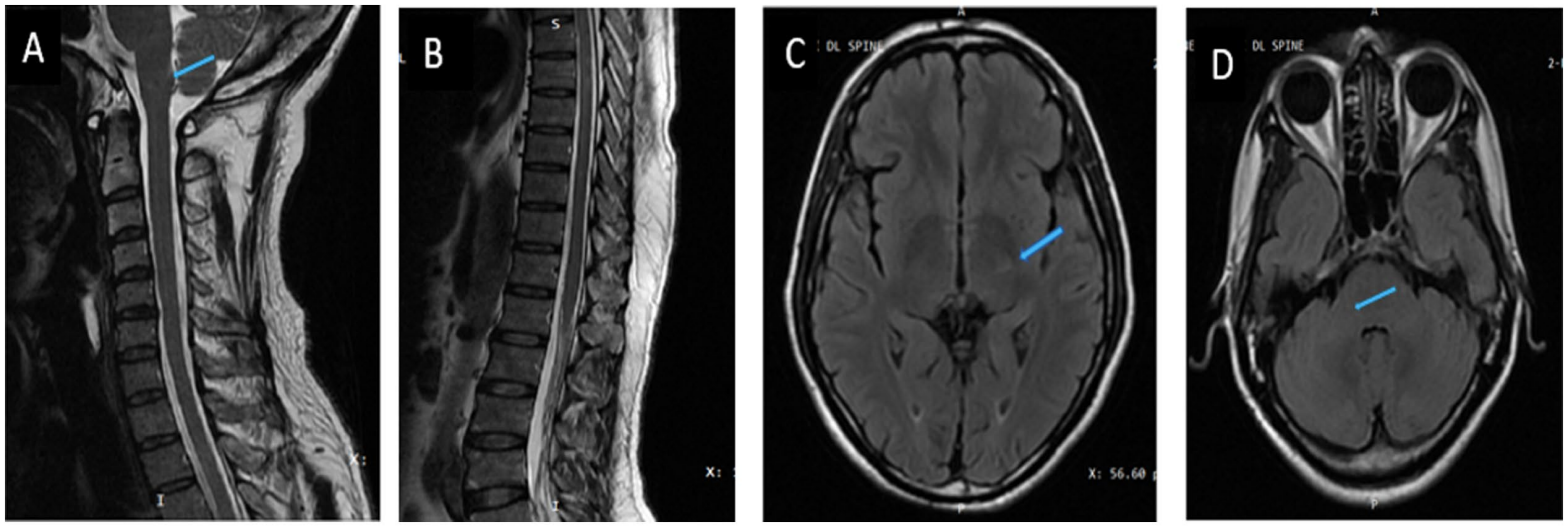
Post-treatment images (6 months after treatment). (A,B) Sagittal T2/STIR image of the cervicodoral MRI. (C,D) Axial T2FLAIR image of brain showing resolution of lesions (blue arrow).
